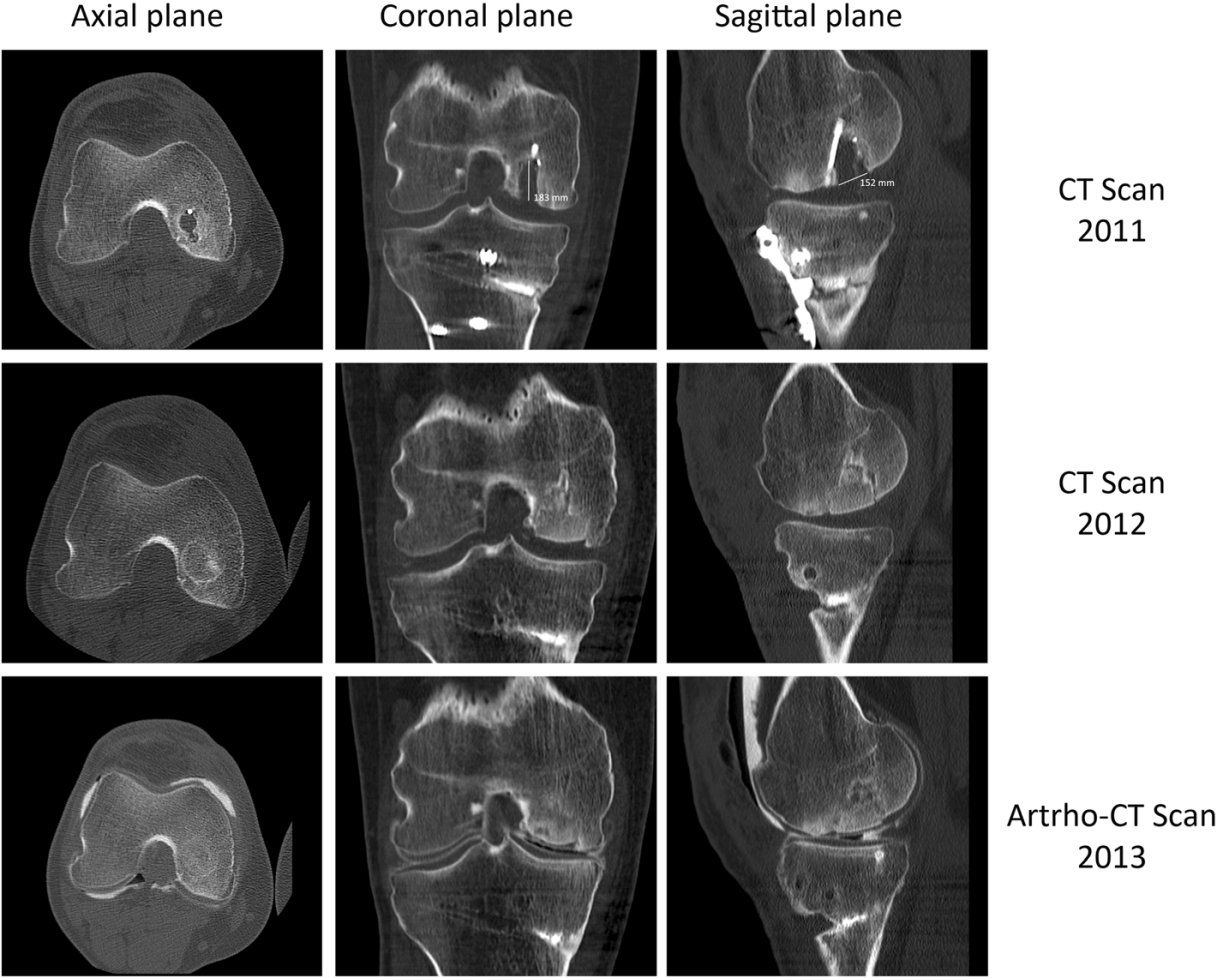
Axial computed tomographic (CT) scan and arthroscopic CT scan follow-up. Images obtained in 2011 show the defect in the femoral condyle where the osteochondral allograft was placed as can be observed in a CT scan obtained in 2012. In 2013, the graft evolved from an area of abnormal intensity signal to a satisfactory reattachment of the graft 2 years after the surgery
